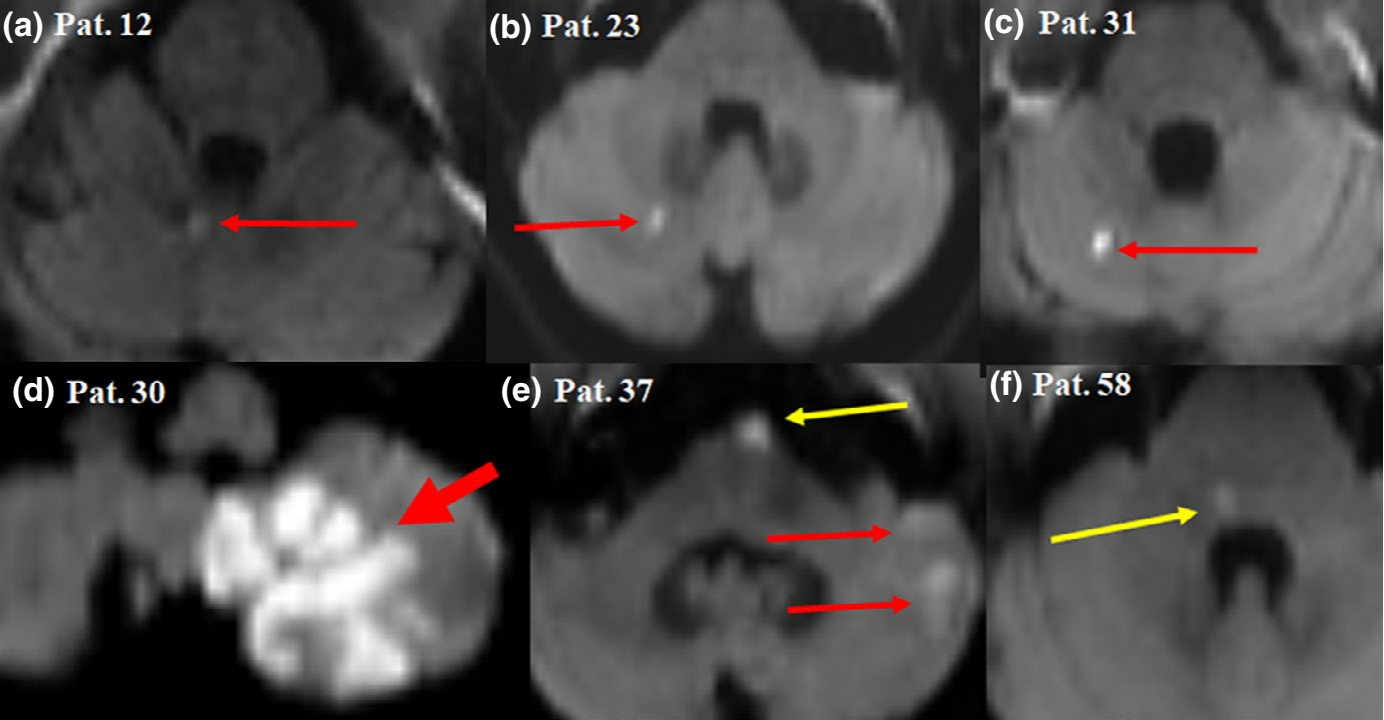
Diffusion‐weighted magnetic resonance imaging showing examples of patients with vertebrobasilar stroke without vertigo. (a) Minute infarction in the vermis (long thin arrow), (b) and (c) minute infarction in the cerebellar hemisphere (long thin arrow), (d) large infarction affecting the cerebellar hemisphere and tonsil (short thick arrow), (e) multiple infarctions affecting the ventral pons (long thin yellow arrow) and the cerebellar hemisphere (long thin red arrow), (f) minute infarction affecting the dorsal pons (long thin yellow arrow). Note the small size of the infarctions in comparison with Figure 2
